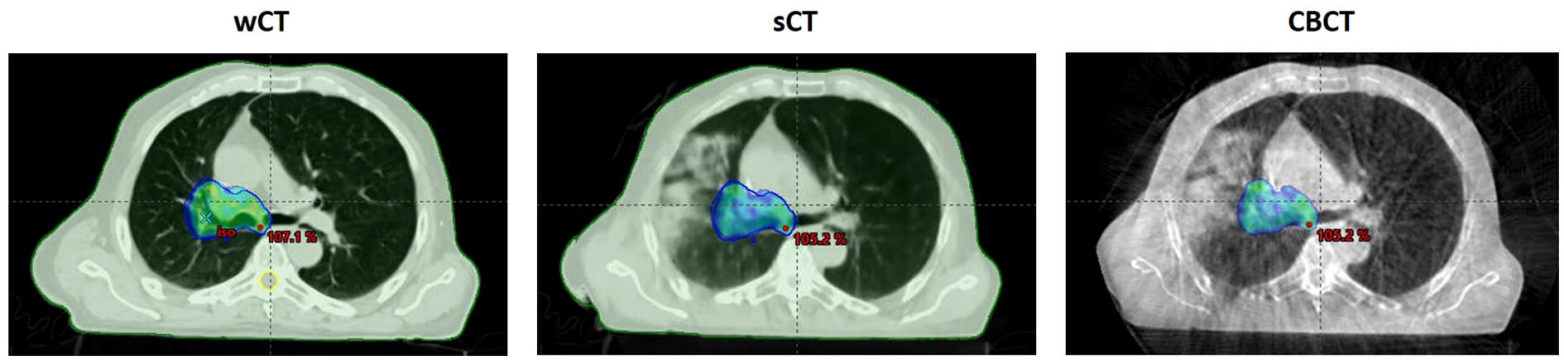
Example of comparison of dose distribution (95% isodose) calculated on the wCT (left), sCT (center) and displayed on the CBCT (right) for a patient treated for a lung cancer.
